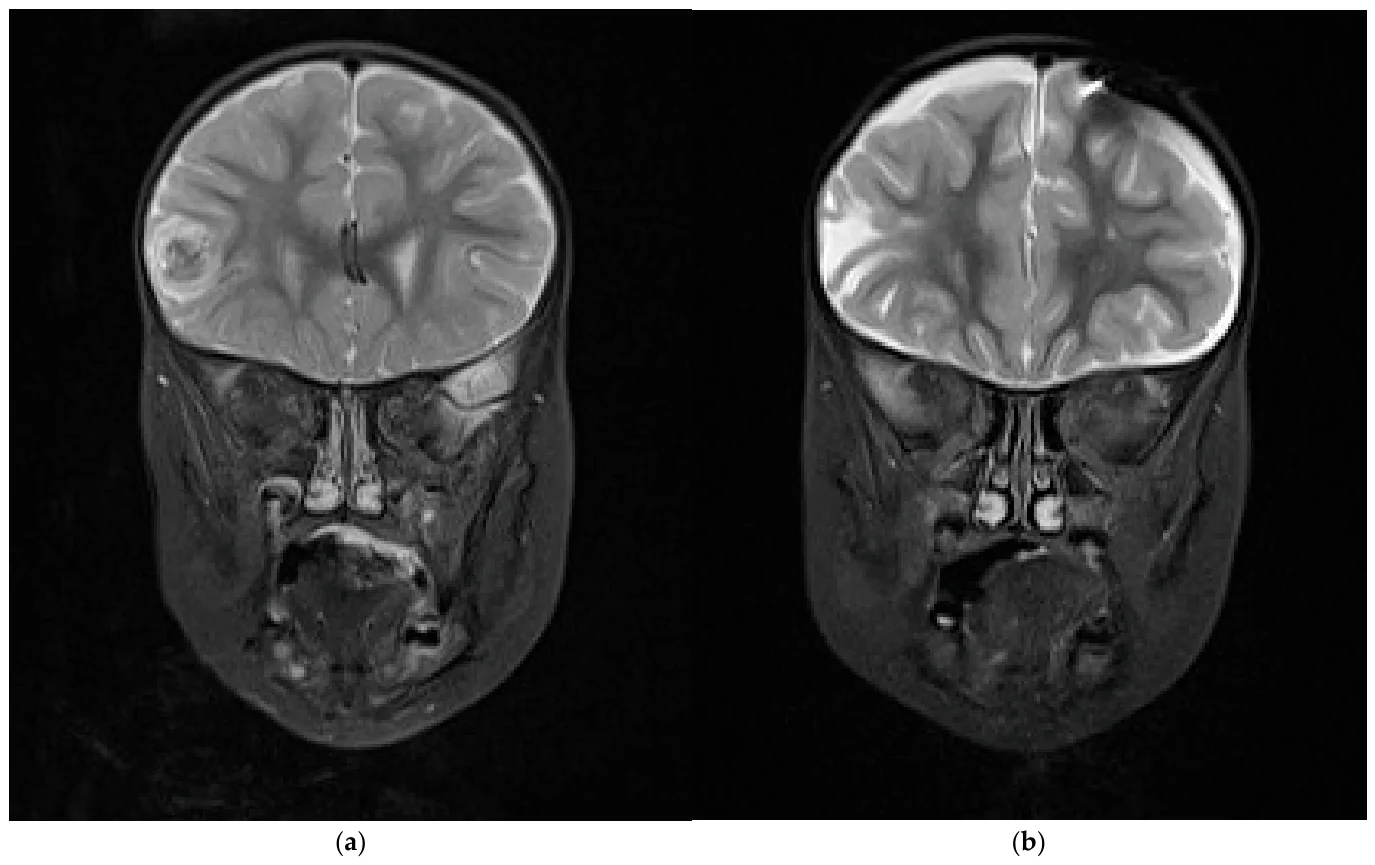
(a,b) Follow-up MRI findings of the cerebral metastasis. (a) Coronal T1-weighted magnetization-prepared rapid gradient-echo (MPRAGE) sequence showing a hemorrhagic metastasis in the right frontal region. (b) Coronal short tau inversion recovery (STIR) sequence demonstrating an unremarkable resection cavity in the right frontal region after surgical treatment. (University Clinic and Polyclinic for Diagnostic Radiology, University Hospital Halle.; Dr. C. Kunze).
